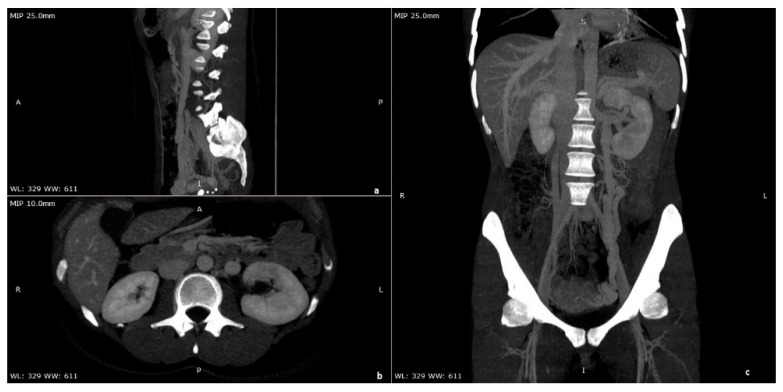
Maximum intensity projection (MIP) = 25 mm and multi-planar reformation (MPR), (a) sagittal, (b) axial, and (c) coronal contrast enhanced CT portal venous phase images of a young woman affected by nutcracker syndrome with pelvic congestion syndrome, acquired in portal venous phase; in (a) and in (c), pathological serpiginous dilatation of the left ovarian vein is shown.
